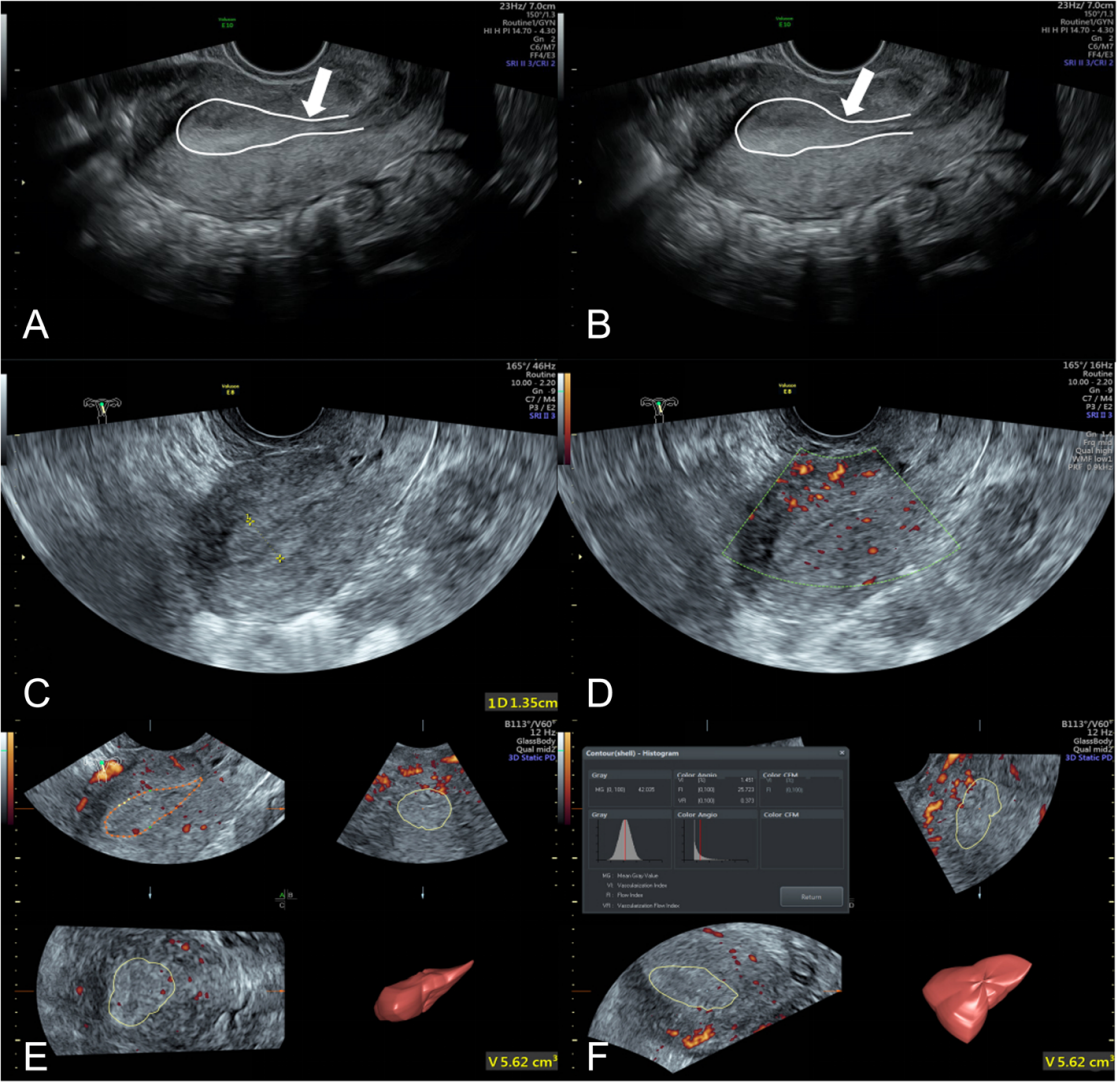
Ultrasound images of a subject in the control group. a, b Two-dimensional gray-scale mode; two images of one peristalsis wave during observation. White curve, outline of endometrium. White arrow, incisura of peristalsis wave. Six positive endometrium peristalsis wave within 3 min (2/min); score, 3; c two-dimensional gray-scale mode; endometrial thickness, 13.5 mm; score, 3; endometrial type, type A; score, 3; d two-dimensional color Doppler mode; endometrial and subendocardial blood flow were observable; score, 3; e three-dimensional mode; endometrial volume, 5.62 cm^3^; score, 3; f three-dimensional mode; VFI, 0.373; score, 3
